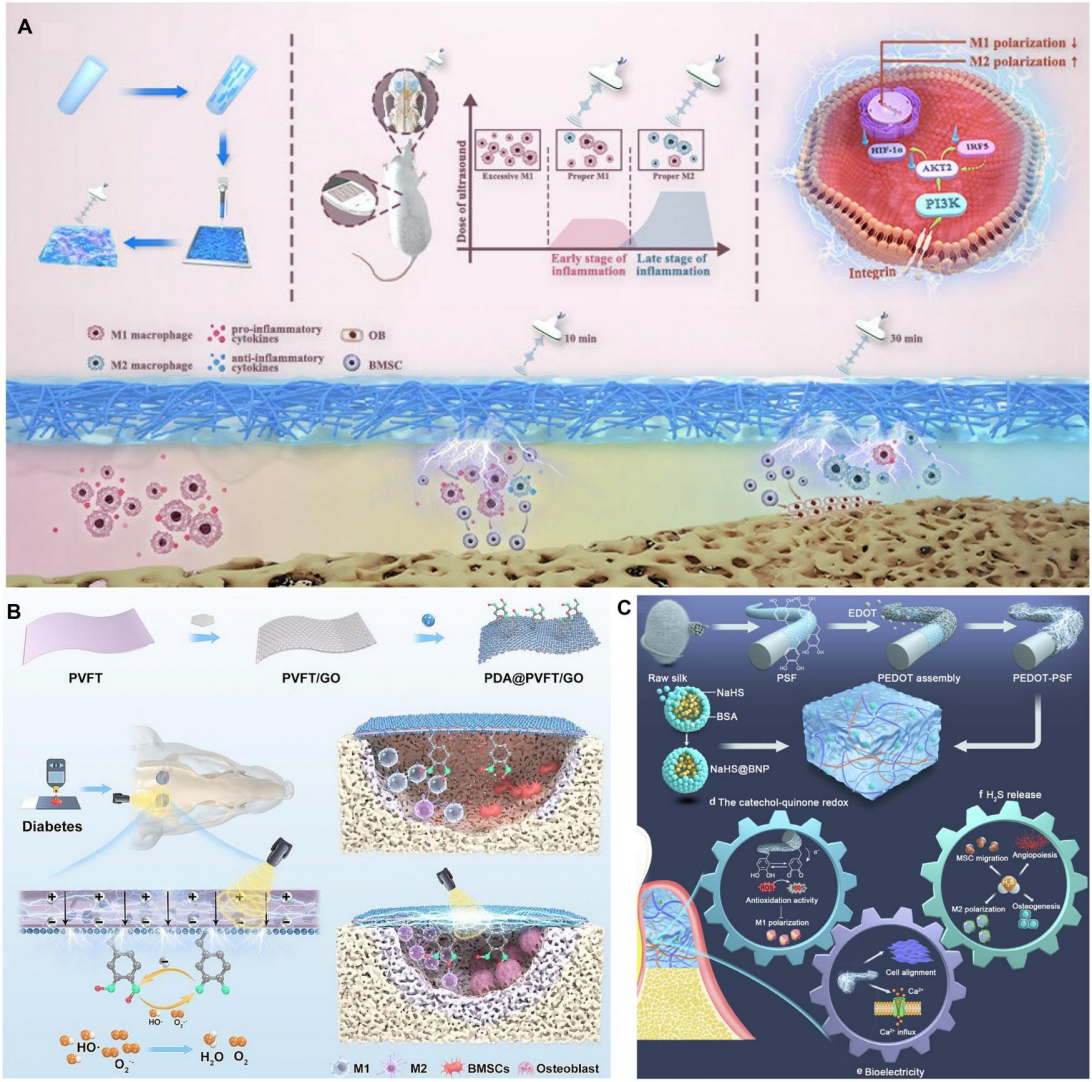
Endogenous signaling mimicking for diabetic bone regeneration. (A) Ultrasound-responsive electroactive membrane generated controlled electrical signals that dynamically reprogrammed macrophage phenotypes via the AKT2-IRF5/HIF-1α axis reproduced with permission from Ref. [207]. Copyright 2023, American Chemical Society. (B) Piezocatalytic P(VDF-TrFE) membrane regenerated its antioxidant capacity under ultrasound stimulation through charge polarization effects. Reproduced with permission from Ref. [208]. Copyright 2024, Elsevier. (C) PEDOT fibers encapsulated NaHS, enabling dynamic redox cycling through electron transfer to promote intracellular antioxidant defenses and inflammation control. Reproduced with permission from Ref. [209]. Copyright 2024, Springer Nature.
